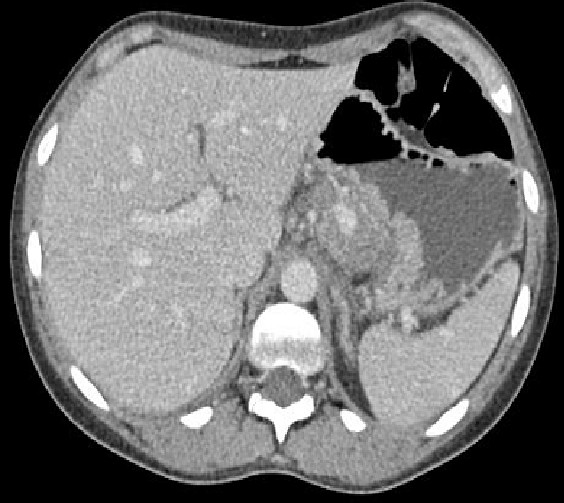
Contrast enhanced CT-scan showing left gastric artery pseudoaneurysm.
